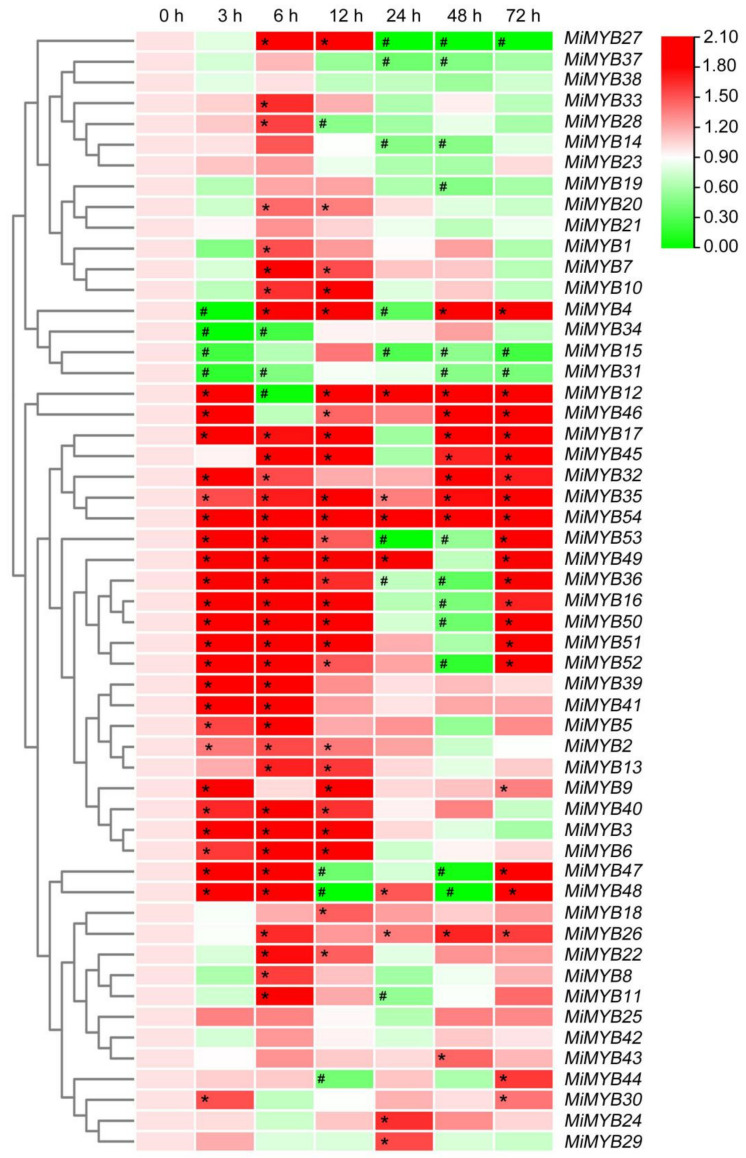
Differential expression of *MiMYBs* in response to H_2_O_2_ treatments. Relative transcript levels of *MiMYBs* in 10 mmol/L H_2_O_2_ treatment at 0, 3, 6, 12, 24, 48, and 72 h. The relative transcript levels of *MiMYBs* in the no-treatment mango leaves (0 h) were normalized to 1.0. The color scale represents gene expression levels with high transcript levels (red) or low transcript levels (green). The calculation method was 2^−∆∆CT^, and ‘*’ means the value exceeds 1.5, while ‘#’ means the value is below 0.5.
